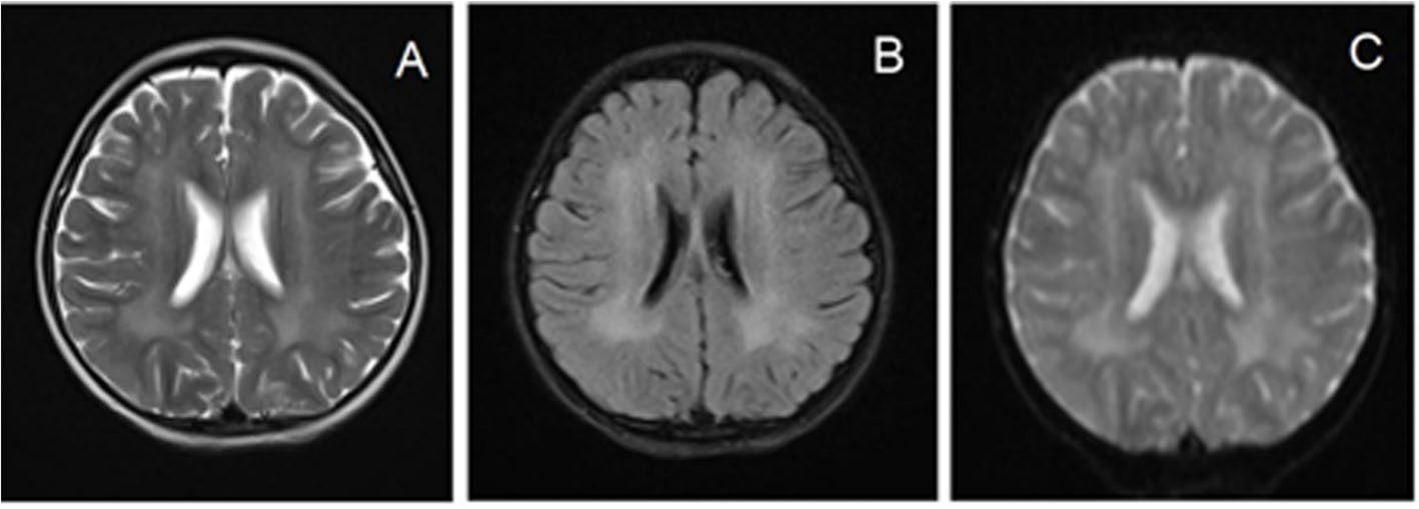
Cranial MRI results of children aged 3 years and 5 months. Images taken by A. T2WI, B. Flair, C. DWI, T2WI and Flair showed that the areas of high signal intensity in the lateral ventricles and patchy areas in the bilateral frontal lobes were larger than those before
